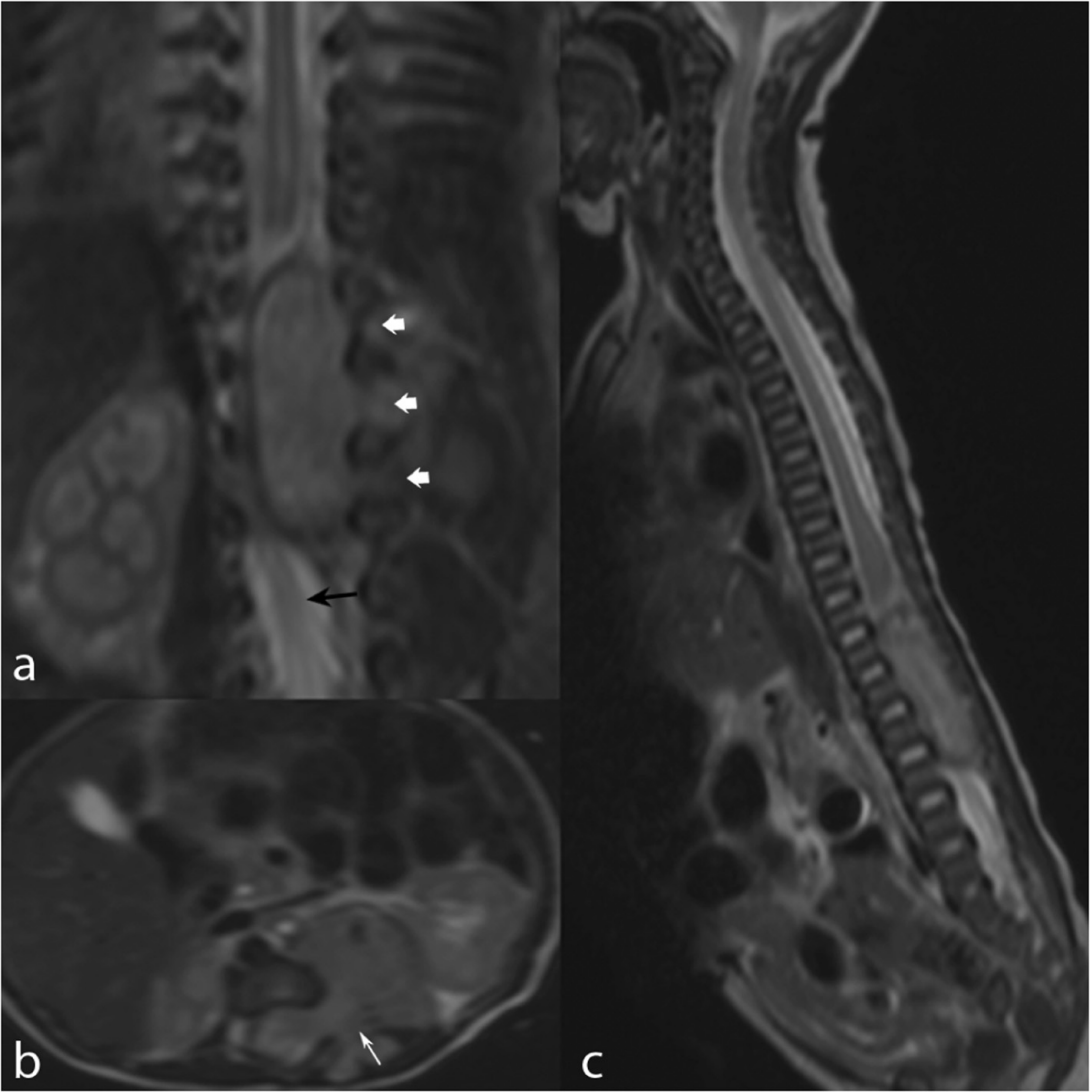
MRI at diagnosis. Three planar T2WI (a, b, c). Retroperitoneal neuroblastoma with intraspinal invasion. Left-sided large mass with dumbbell extension through widened neural foramina (a and b, *white arrows*) and marked compression and dislocation of the cauda (a, *black arrow*)
